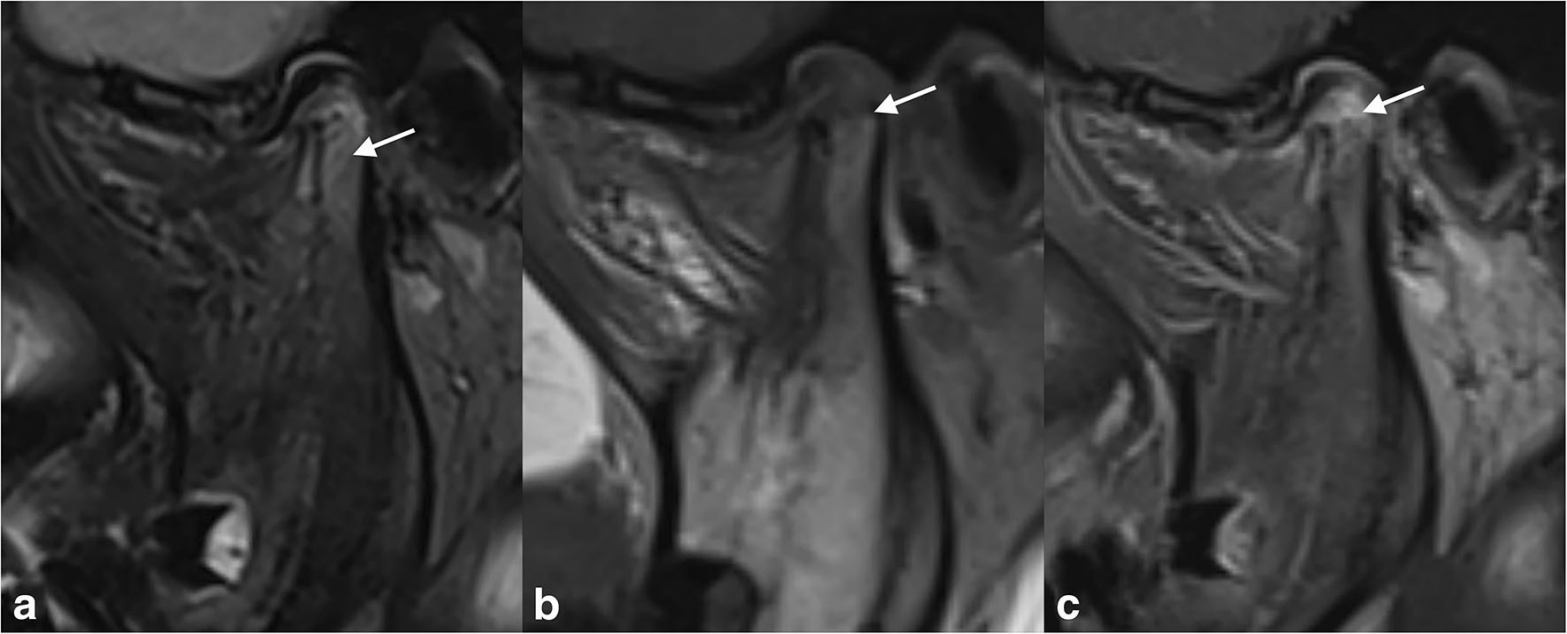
A 13-year-old girl with JIA and active TMJ arthritis. MRI of the TMJ with (a) a sagittal oblique T2-weighted fat-saturated image showing bone marrow oedema (hyper-intense signal on T2-weighted images (arrow)) in the TMJ condyle, (b) a sagittal oblique T1-weigted image showing bone marrow oedema (hypo-intense signal on T1-weighted images) and condylar flattening (arrow) and (c) a sagittal oblique T1-weighted fat-saturated post-Gd image showing joint and condylar enhancement (arrow)
